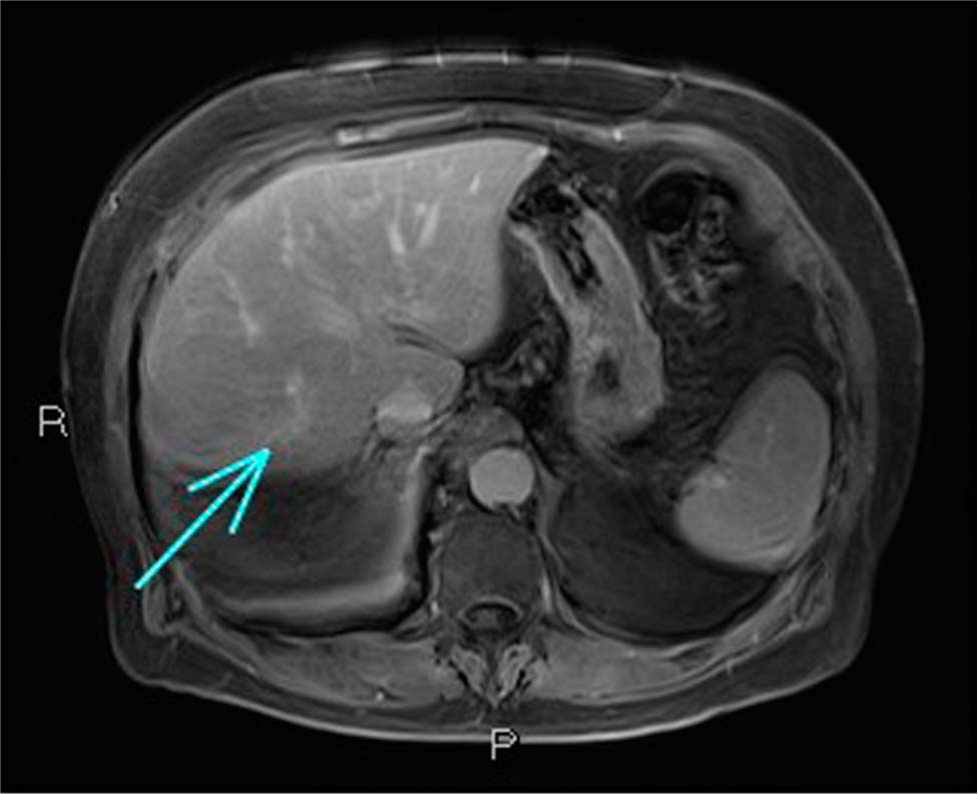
Magnetic resonance imaging at 18-month mark; enhancing scar at the site of previous lesion depicted by the arrow.
